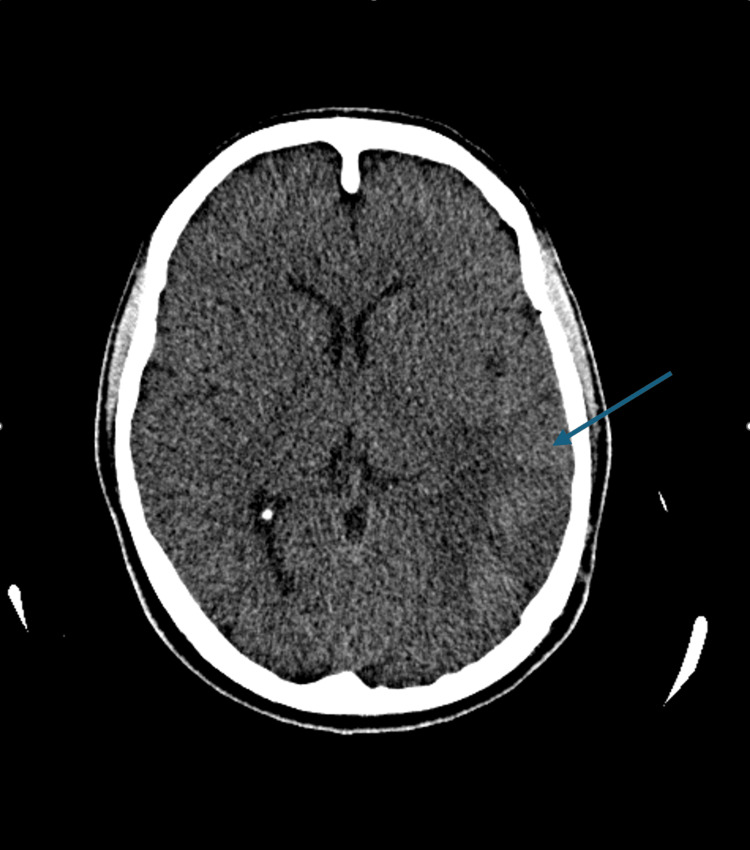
CT brain plain axial image showing left parietotemporal hemorrhage (blue arrow).
